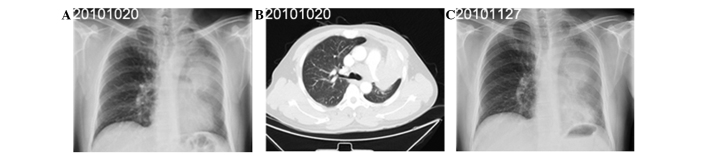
Case two: Representative images from radiography and chest CT revealing the presence of a mass in the upper lobe of the left lung and enlarged lymph nodes. (A) Radiograph prior to treatment. (B) Representative CT image prior to treatment. (C) Radiograph after one cycle of first-line chemotherapy treatment. CT, computed tomography.
